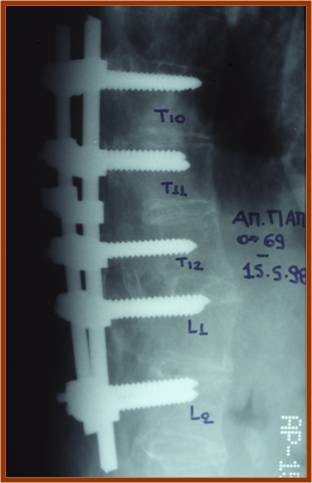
Lateral postoperative radiograph of the same case.
